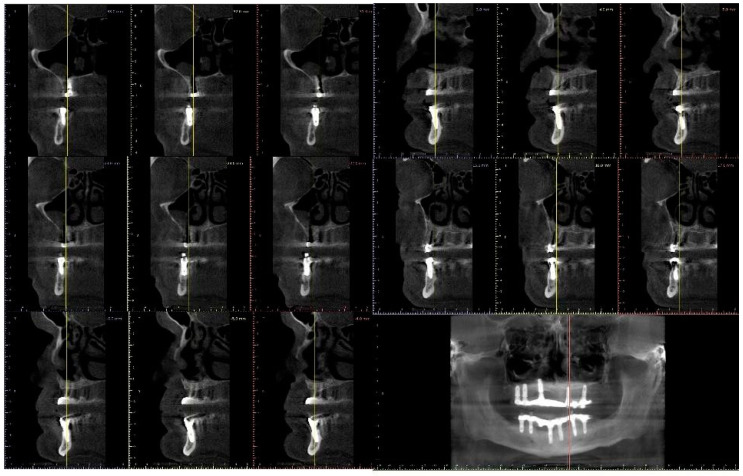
CBCT Cross section projection of the clinical case at the follow up.
